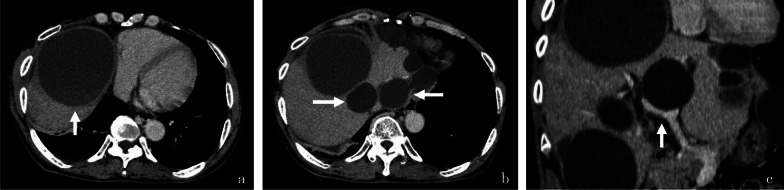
Computed tomography (CT) image taken at the first visit. The cyst is observed in the eighth segment of the liver and measures 13 cm in diameter; it is the largest of the cysts in this case. The cyst appears to have compressed the right side of the heart and the right thoracic cavity (a). The cysts are observed to be located dorsal to the liver on both sides of the inferior vena cava (IVC); they were thought to have caused the stenosis of the IVC (b). One of the cysts is seen to be in contact with the right and left branches of the portal vein; the right branch appears compressed and stenotic (c)
